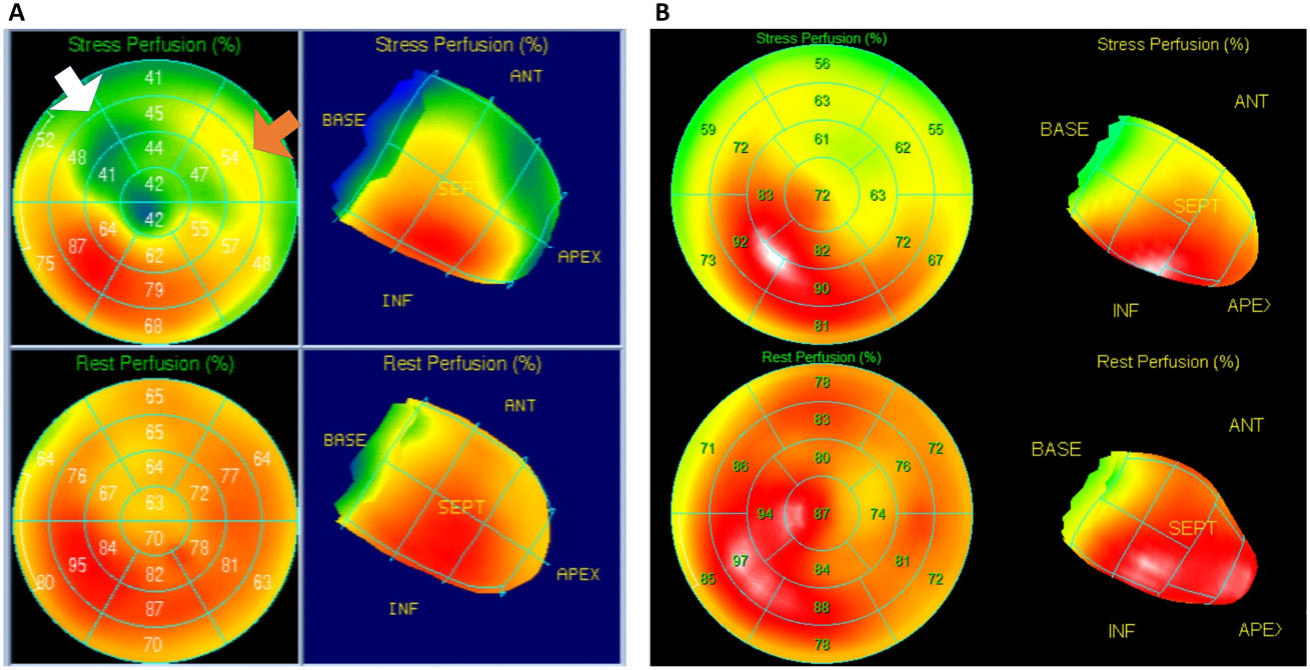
Results of preoperative and postoperative Quantitative Perfusion SPECT in Case 4. (A) Preoperative Tc-TF scintigraphy. This examination documents a reversible perfusion defect at rest (bottom rows) and during stress (top rows). The polar plots depict the extent of the ischaemic area in the anterior (white arrow) and lateral left ventricular walls (brown arrow). (B) Postoperative TI scintigraphy. Perfusion polar maps during stress (top rows) and at rest (bottom rows) show remarkably improved myocardial perfusion. SPECT: single-photon emission computed tomography; Tc-TF: technetium-tetrofosmin; TI: thallium.
